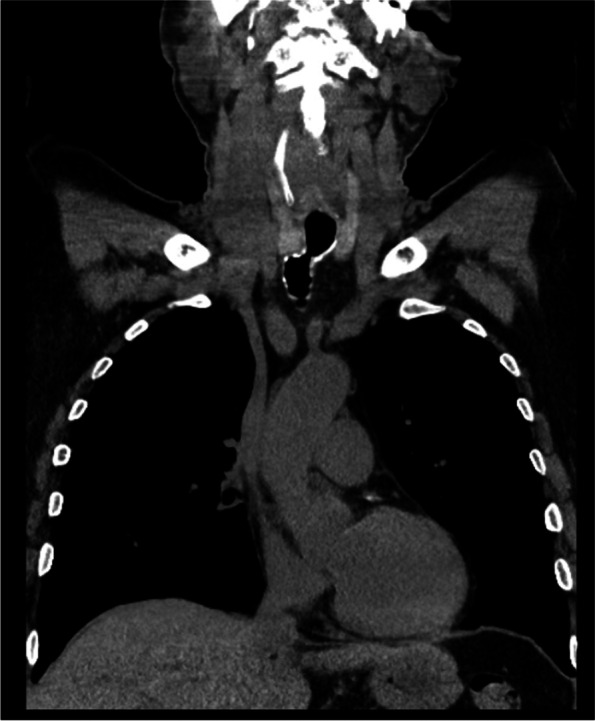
Coronal CT scan. The stenotic segment measured 1.8 cm in length, with a minimal medial-lateral diameter of 0.5 cm and minimal AP diameter of 1 cm measured on the coronal and sagittal inspiratory images
